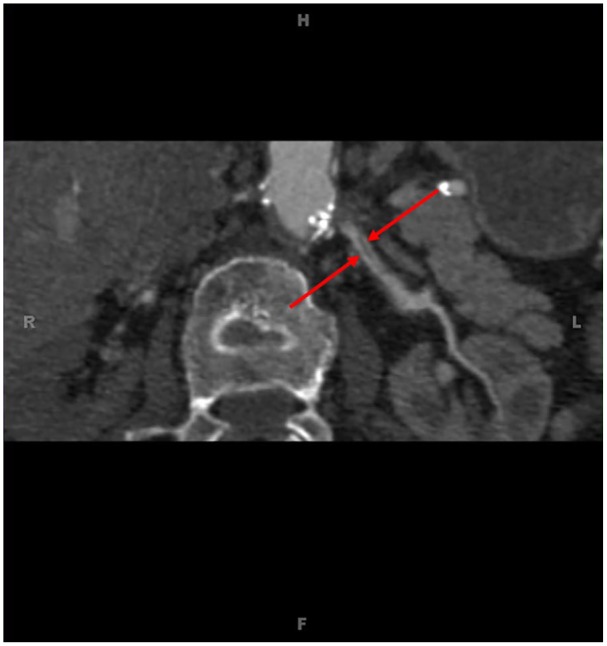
Computed tomography angiogram of the abdomen and pelvis and left kidney showing 2 renal arteries, a superior and inferior. It was determined that the inferior renal artery was 90% stenosed at the origin.
